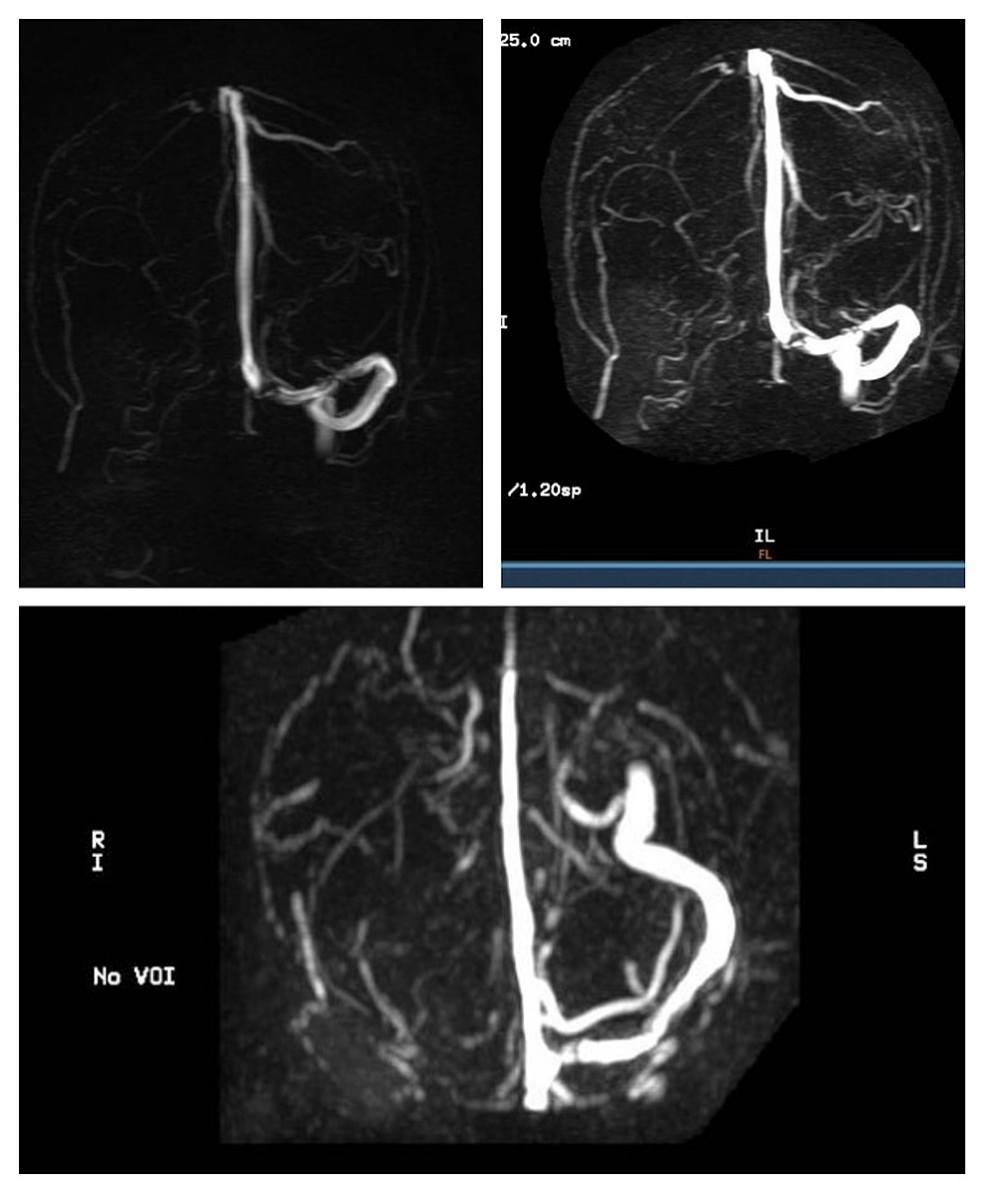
MRI of the brain with MRV revealed massive cerebral venous thrombosis involving sigmoid, lateral, and jugular venous sinuses MRV: Magnetic resonance venography
